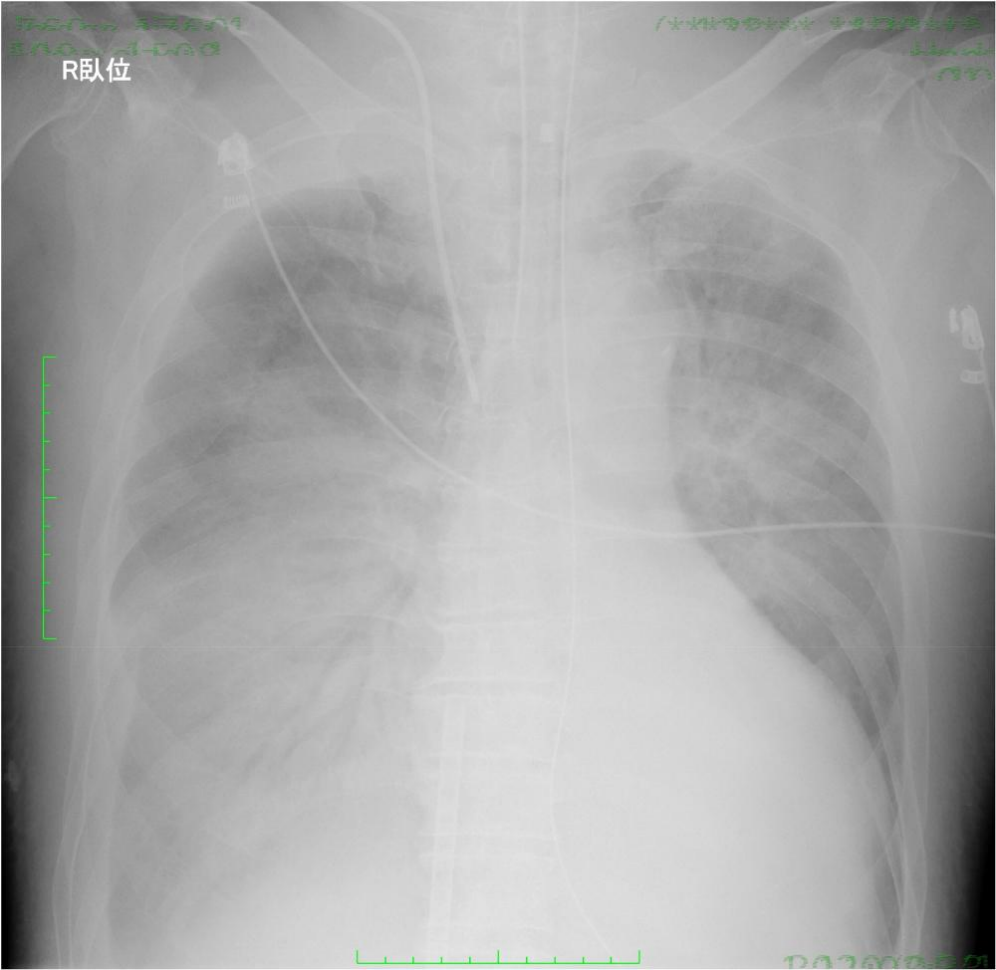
Chest radiograph revealed minimal air content in the bilateral lung fields.
